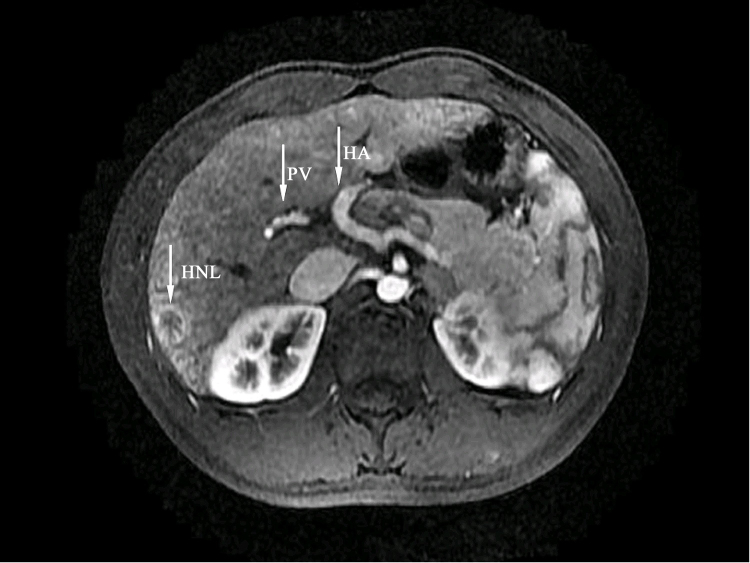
Abdominal magnetic resonance image showing the slender portal vein, rich arterial blood supply and hepatic mass lesion. HA, hepatic artery; PV, portal vein; HNL, hepatic nodular lesion.
